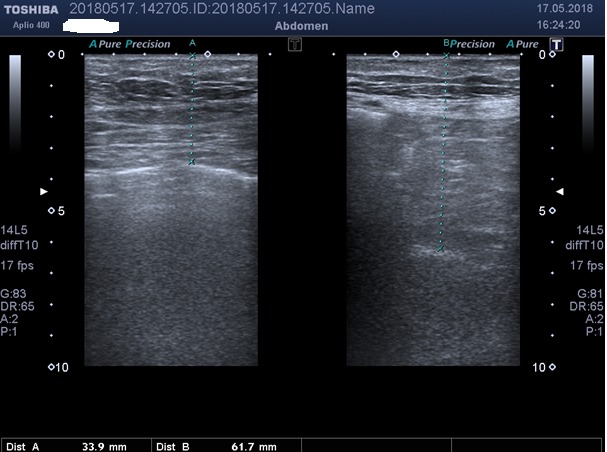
The longitudinal distance the viscera travelled as visualized on ultrasonographyfrompoint A topoint B
